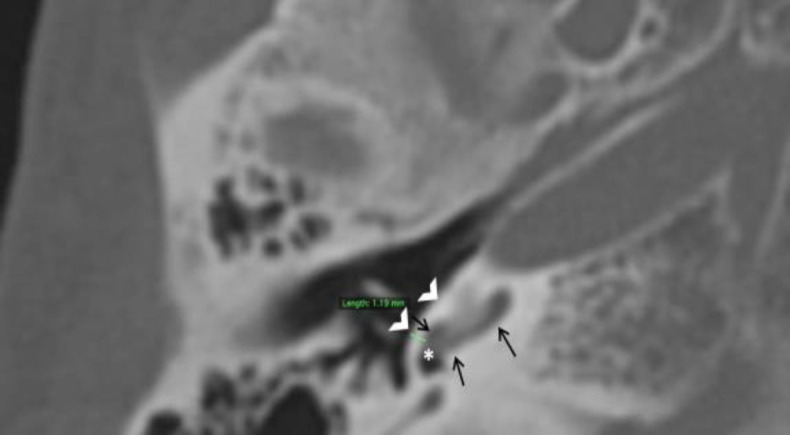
Axial CT image of temporal bone demonstrating method used to measure thickness of promontory (PT) as depicted by straight green line. Note that the measurement corresponds to lateral bony wall of basal turn of cochlea (black arrows) adjacent to the round window (asterisk). Promontory is indicated by white open arrow heads
